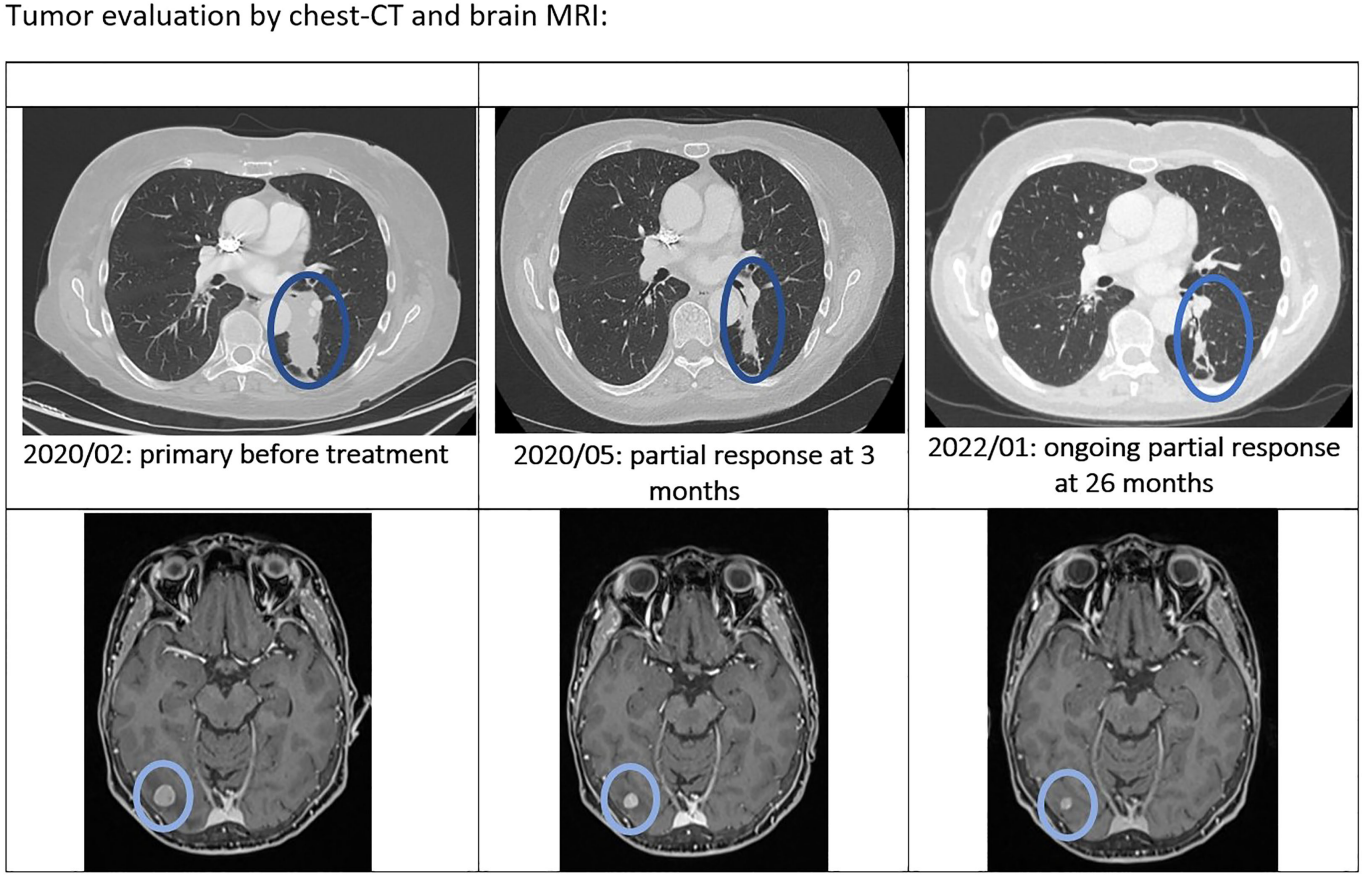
Tumor evaluation by chest-CT and brain MRI.
